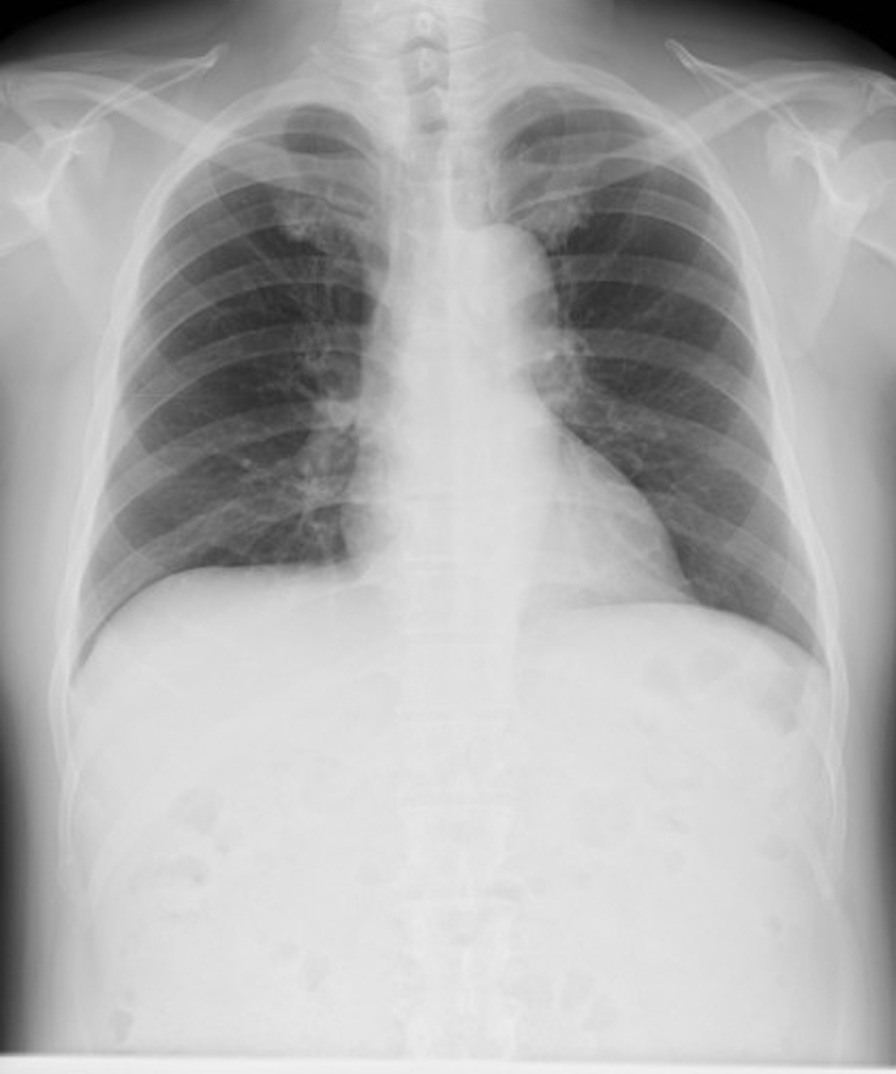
Chest X-ray 4 months prior to myocardial infarction. Normal findings are obsreved
